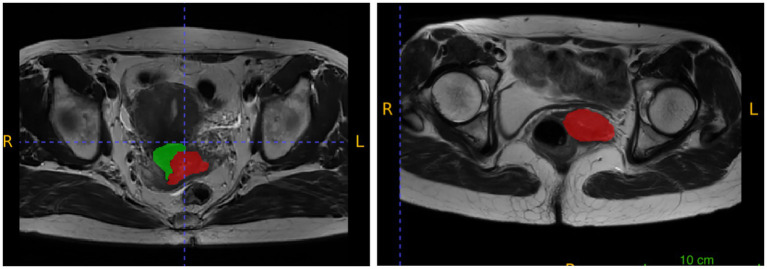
Example of MRI images annotated by the ITK-SNAP software.
